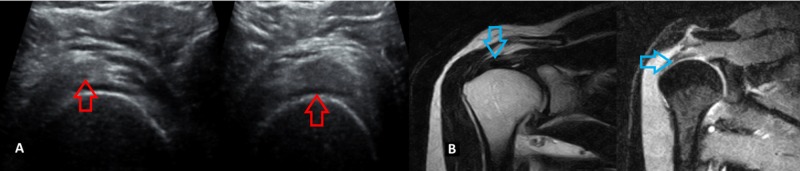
A 40-year-old male patient with supraspinatus tendinosis Longitudinal HRUSG image of the supraspinatus tendon reveals a bulky and heterogeneous tendon with joint effusion (A: red arrow). T2 and STIR coronal MRI image of the shoulder joint reveals an increased thickness with increased signal intensity on coronal T2 and STIR images (B: blue arrow). HRUSG - high-resolution ultrasonography; STIR - short TI Inversion Recovery
